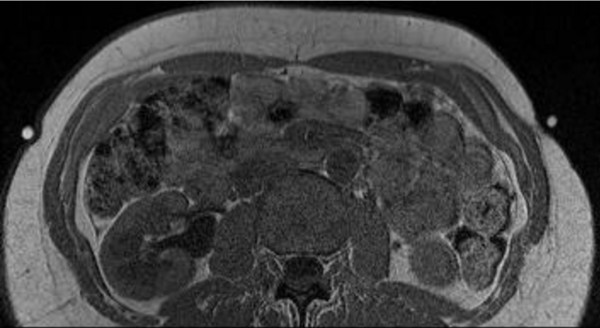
MRI demonstrating re-grown subcutaneous fat tissue at sites of previous lipoatrophy. Axial T1-weighted MRI sequence (1.5 Tesla Magnet, body surface coil, slice thickness 3 mm) acquired through the abdomen, March 2010. Markers are placed over the healed lipoatrophic area (left side), and over the biopsy scar (right side), respectively. Signal intensity and subcutaneous tissue structure indicate re-grown subcutaneous fat tissue at the healed area (left side; high signal intensity, identical to that of periumbilical subcutaneous fat tissue), and fibrous tissue at the biopsy area, respectively (right side; signal intensity is only half of that of subcutaneous fat tissue).
